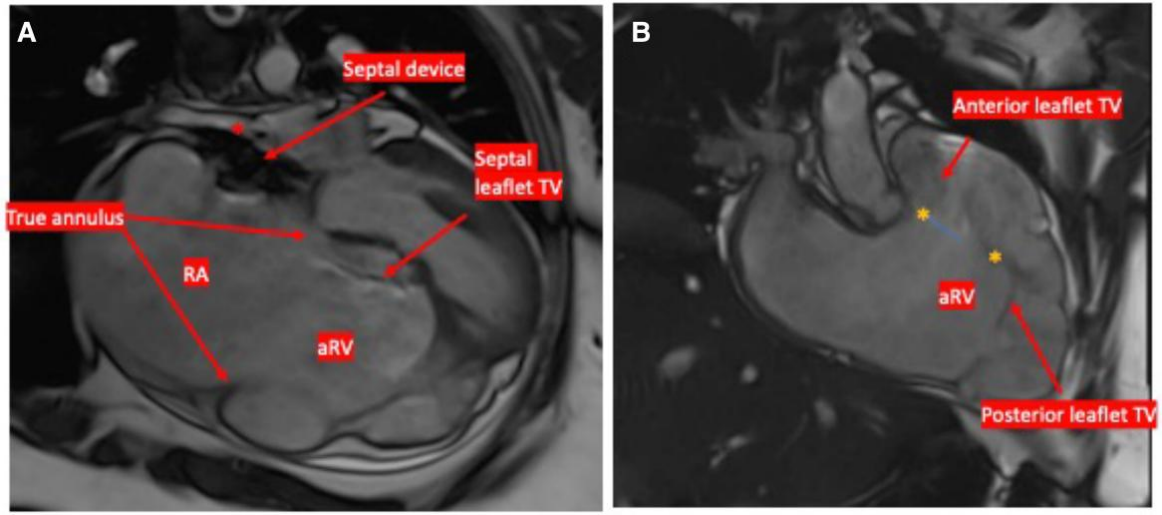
Cardiac magnetic resonance imaging four-chamber view (*A*) and RV inflow–outflow view (*B*) in end diastole demonstrating dilated atrialized inlet of the right ventricle with small functional right ventricle; the distance between the true tricuspid valve annulus and functional tricuspid valve orifice (in yellow asterisks) defines the atrialized inlet of the right ventricle, compressed right lower pulmonary vein denoted by the star (*) next to the septal occlusion device. RA, right atrium; RV, right ventricle; aRV, atrialized inlet of RV; TV, tricuspid valve.
